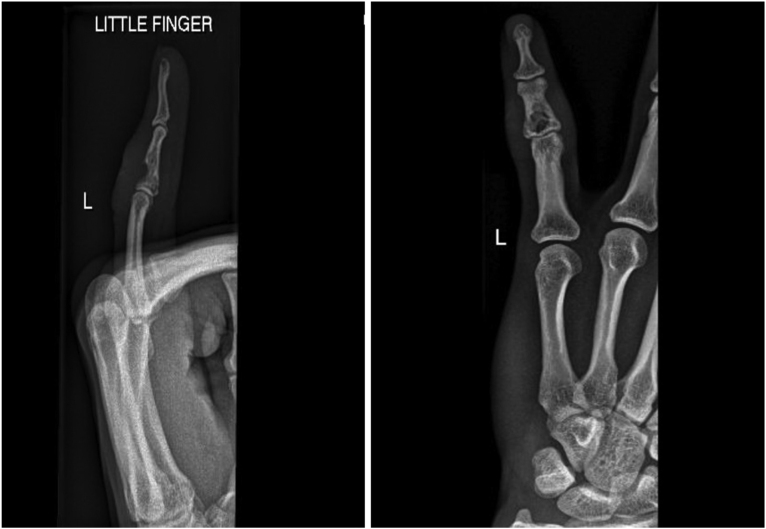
X-rays of the left little finger demonstrating middle phalanx osteomyelitis with no joint involvement.
